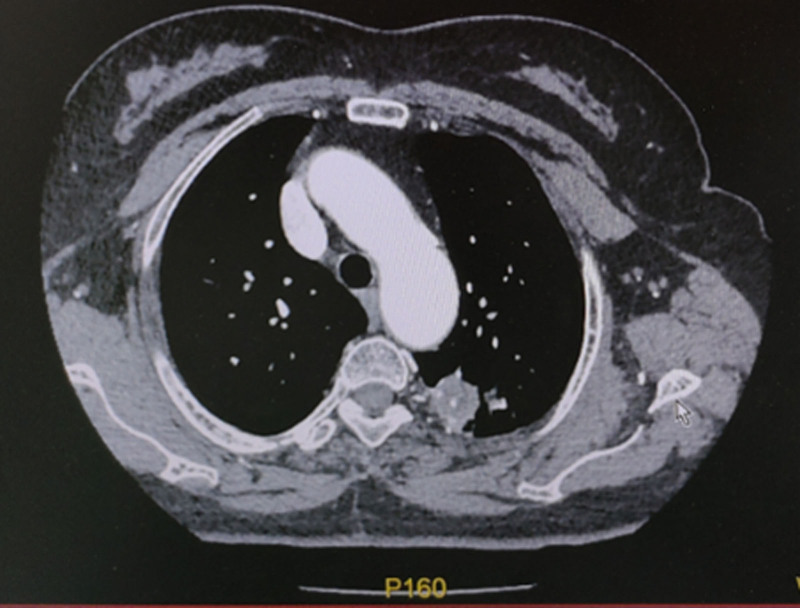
Chest enhanced CT scan. An enhanced scan (Fig. 3) showed uneven enhancement of the upper left lung mass. CT = computed tomography.
